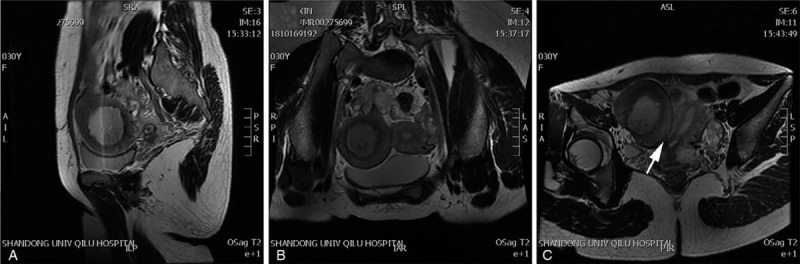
MRI showing uterine dysplasia (right rudimentary uterine horn and left unicornate uterus) and hematometra of the reported case. A: hematocele in rudimentary horn uterine cavity. B: right rudimentary horn uterine with hematocele and left unicornate uterus. C: no passage between rudimentary horn and cervix or unicornate uterus (arrow).
